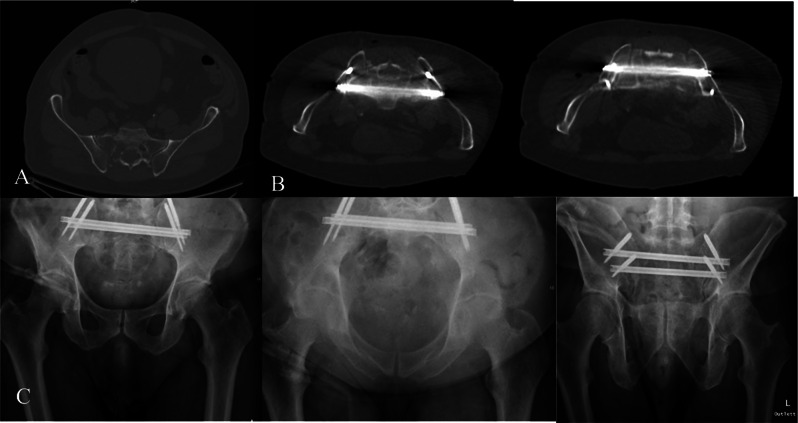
Patient 3 A- preoperative CT scan showing sacral fracture, B- intraoperative CT scan, C- X- Rays demonstrating no implant failure 4 months postoperative
